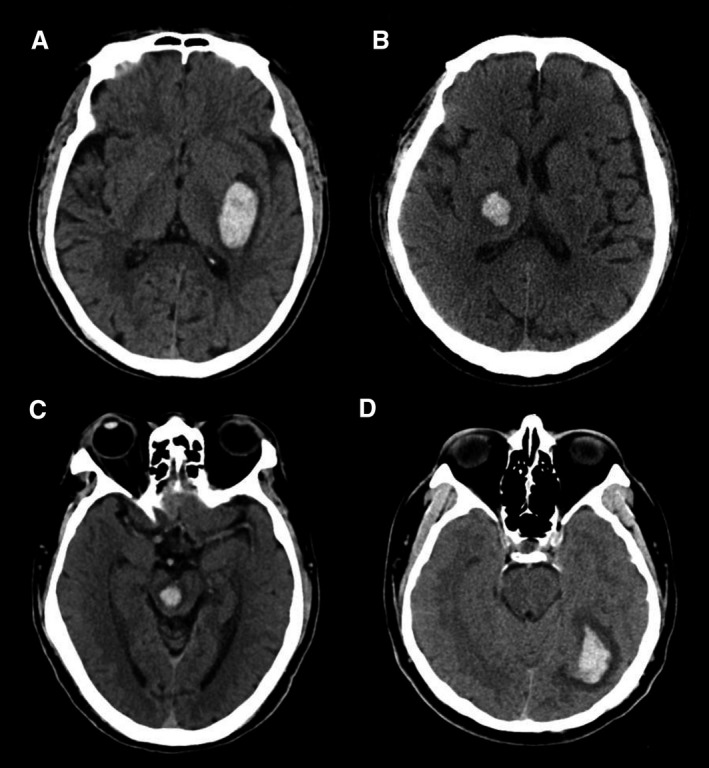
Representative images of benign intracerebral hemorrhage (ICH). Representative axial noncontrast computed tomographic images in patients with benign ICH. Basal ganglia (A), thalamic (B), brainstem (C), and lobar benign (D) hemorrhages. Benign hematomas are relatively regular in shape and homogeneous in density.
